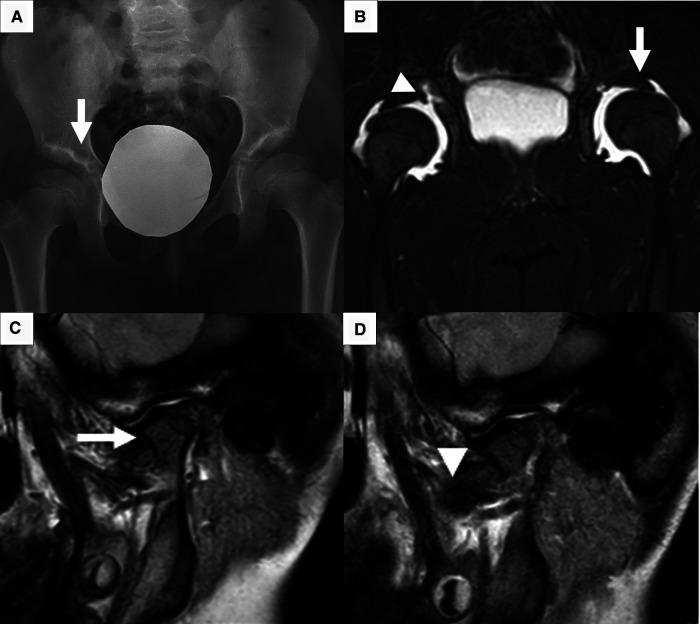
Main radiological feature. (A) X-ray of the pelvis shows flattening of femoral heads, stubby and short femoral neck, and bilateral coxa vara. Acetabular roofs are irregular with sclerotic surfaces and radiolucent lytic areola (*arrow*) as per acetabular cyst. (B) Short tau inversion recovery sequence of pelvis MRI shows effusion at coxofemoral joints resulting in partial femoral head dislocation (*arrow*); acetabular cyst connected with joint effusion (*arrowhead*). (C, D) MRI of left TMJ in close and open mouth shows little/no excursion of the condyle. The condyle has an enlarged and flattened morphology (*arrow*) with irregular surface. A slim layer of intra-articular effusion is present. The articular disc (*arrowhead*) has normal morphology and signal.
